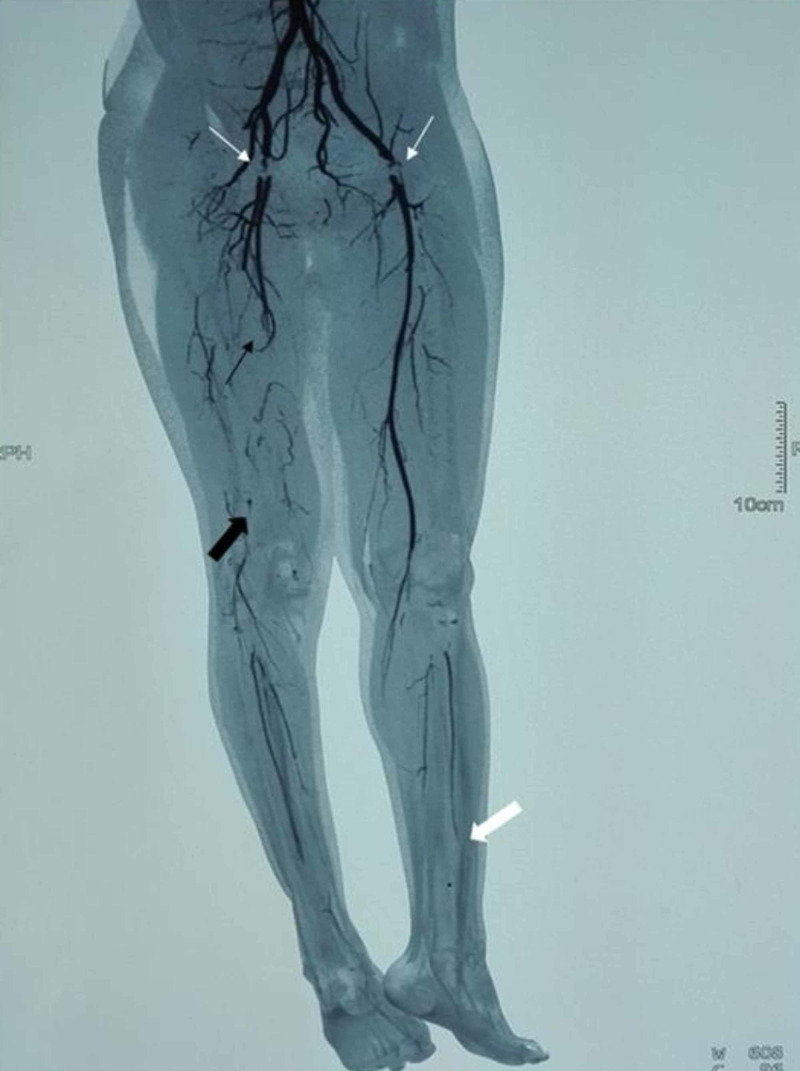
CT arteriography of lower limb reconstructed image Filling defects are visible throughout the length of common femoral arteries bilaterally (thin white arrows). A filling defect is evident in distal half of right superficial femoral artery (thin black arrow) and whole length of right popliteal artery (thick black arrow). Non-opacification of proximal part of left profunda femoris and left superficial femoral artery, and normal flow in the distal parts of these arteries is evident. In the left limb, anterior tibial artery is attenuated distally (thick white arrow) and dorsalis pedis is absent.
